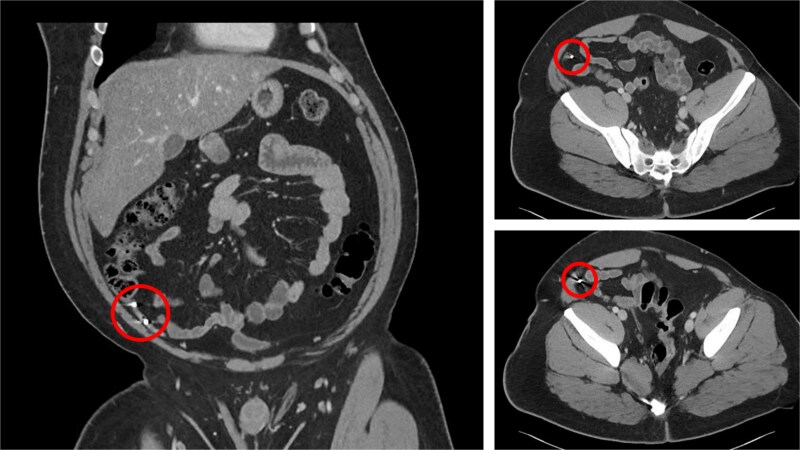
CT of the abdomen of the patient.
